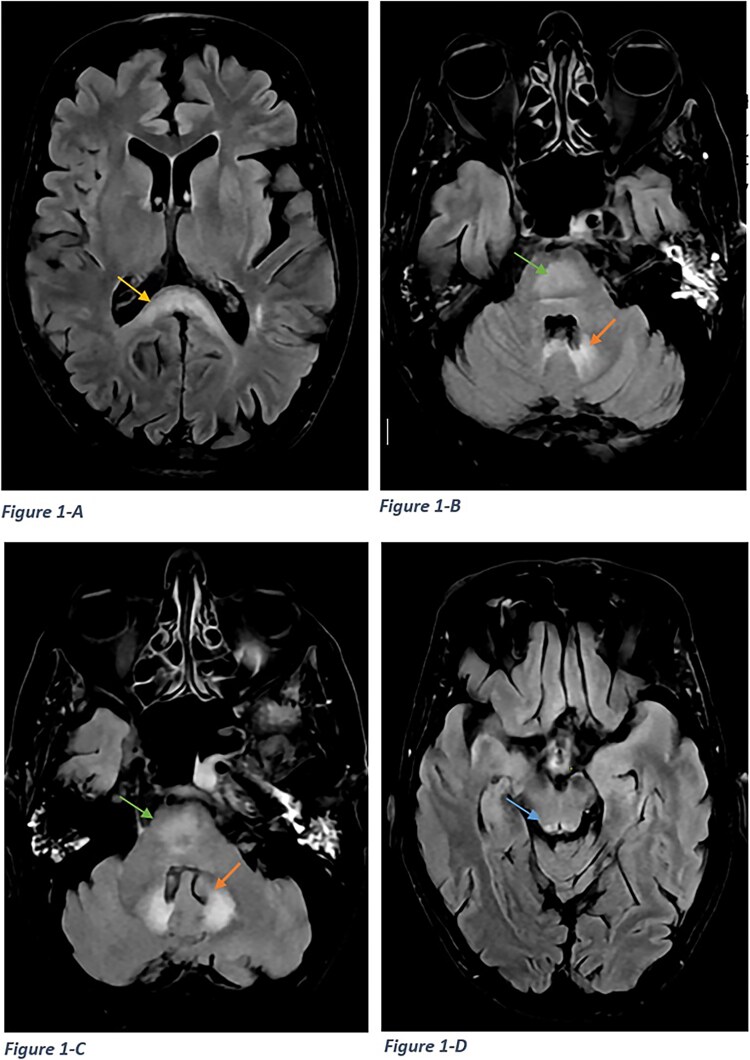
Axial MRI Flair sequence showing high signal in the splenium of the corpus callosum (A), dorsal pons (B-C), bilateral dentate nuclei (C), and tectum (D).
